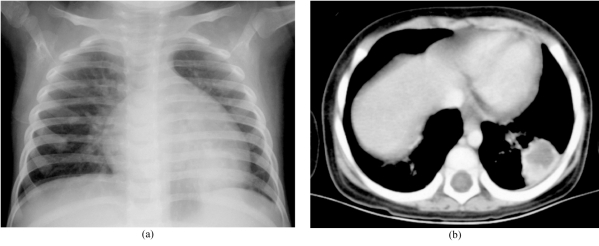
An 18-month-old girl with leukaemia and Aspergillus isolated from the lung. (a) Chest radiograph showed a round opacity behind the heart. (b) CT revealed a cavitating nodule in the left lower lobe.
